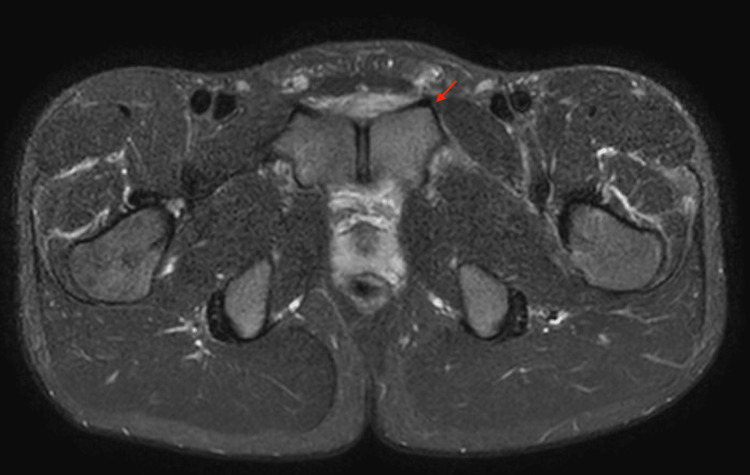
Pelvic magnetic resonance imaging on day three after onset.
